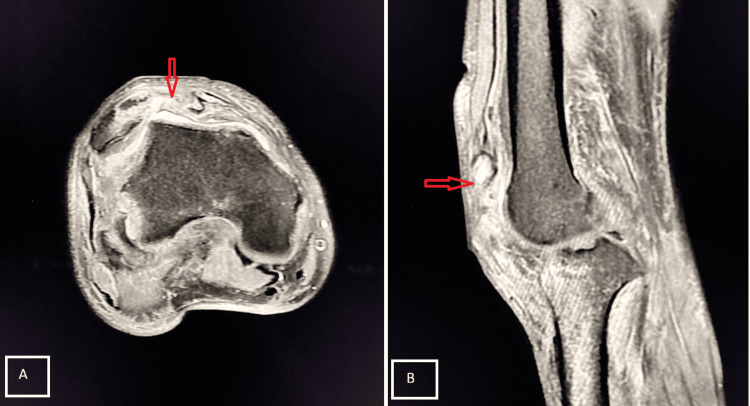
Transverse fracture of the patella Axial (A) and sagittal (B) PD-FS MRI demonstrate a transverse fracture of the patella with marrow edema, associated with mild joint effusion and surrounding soft tissue edema (red arrow). PD-FS, proton density fat-suppressed
